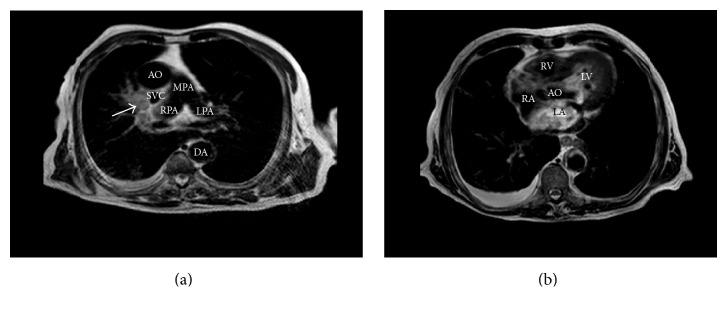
Chest magnetic resonance imaging showing invasion and subtotal obliteration of left atrial cavity from the right upper pulmonary vein (arrow). AO, aorta; DA, descending aorta; LA, left atrium; LPA, left pulmonary artery; LV, left ventricle; MPA, main pulmonary artery; RA, right atrium; RPA, right pulmonary artery; RV, right ventricle; SVC, superior vena cava.
